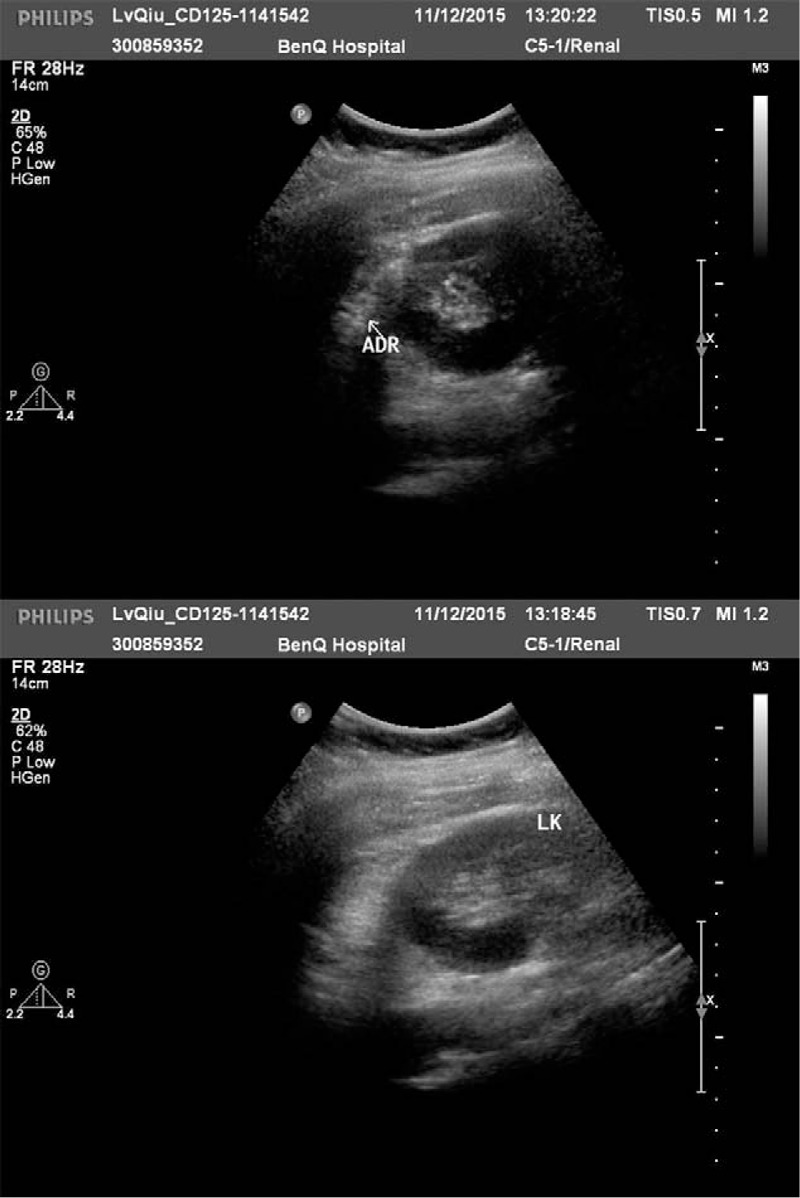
Ultrasound demonstrated the normal shape of adrenal gland (white arrow), kidney and pancreas. The cyst disappeared after surgery.
